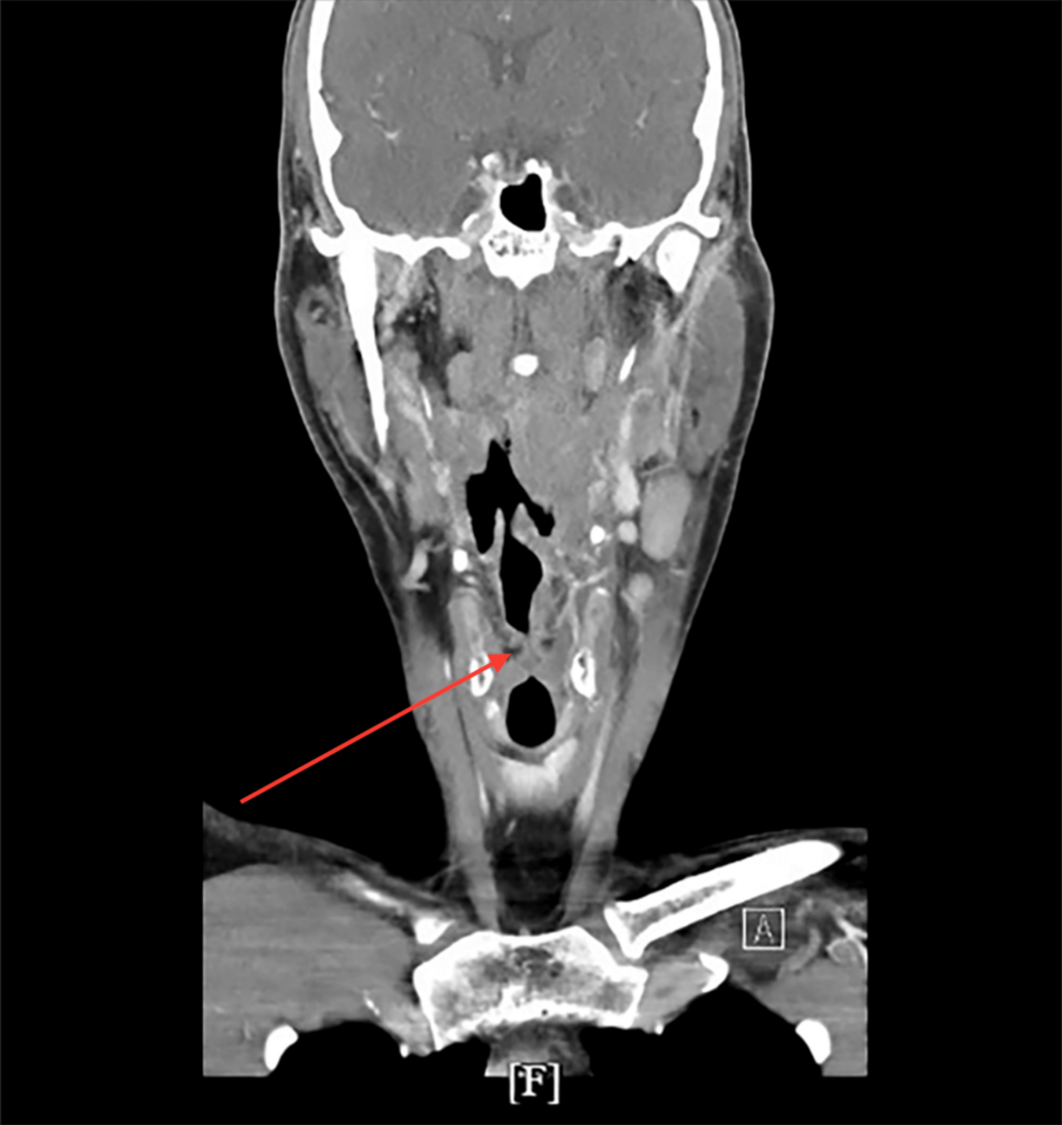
Contrast-enhanced coronal CT of the neck demonstrating thickening vocal cords and walls of the larynx with narrowing of the airway indicated by arrow.
